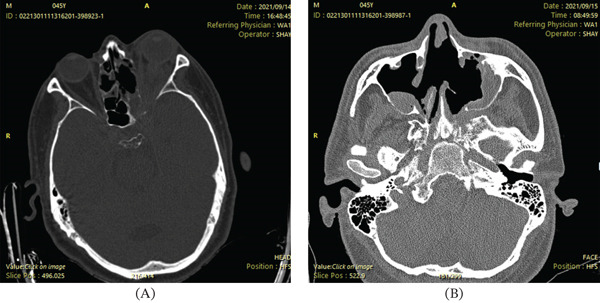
(A) Axial section of orbital compound tomography (CT) scan without contrast demonstrates intraorbital invasion in the left eye. (B) Axial section of paranasal sinuses CT scan shows evidence of paranasal sinuses debridement and collection in both maxillary sinuses.
